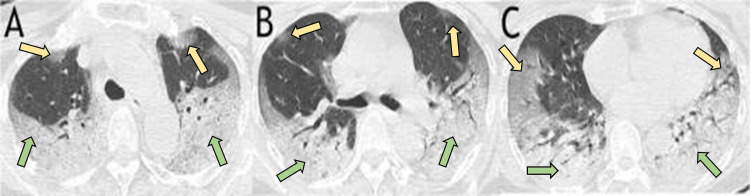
CT images showing various extents of consolidation and areas of GGO within both lungs The CO-RADS score was calculated as: for upper zone [A], 3 [consolidation] x 3 [50-75% distribution] x 2 [both right and left lungs] + 2 [ground glass opacity] x 1 [< 25% distribution] x 2 [both right and left lungs] For middle zone (B), 3 [consolidation] x 2 [25-50% distribution] x 2 [both right and left lungs] + 2 [ground glass opacity] x 2 [25-50% distribution] x 2 [both right and left lungs] For lower zone (C), 3 [consolidation] x (2 [25-50% distribution of the right lung] + 3 [50-75% distribution of the left lung]) + 2 [ground glass opacity] x (2 [25-50% distribution of the right lung] + 1 [< 25% distribution of the left lung]). Image source: Reference no. [16]
